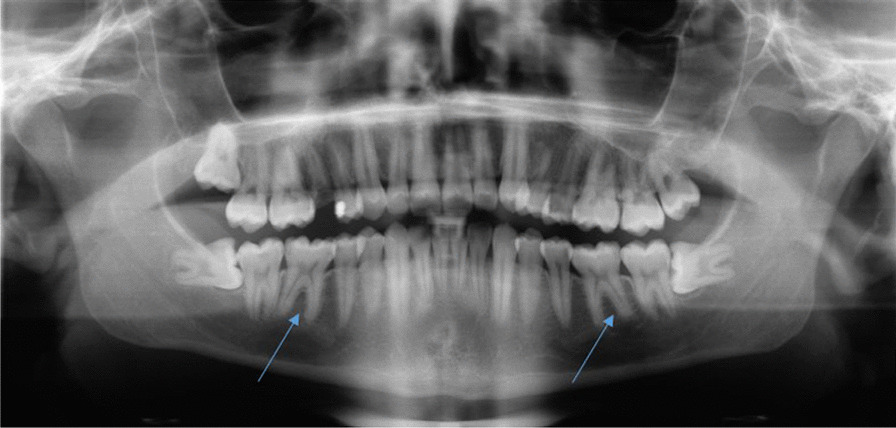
Routine X-Ray taken in January 2019 by the primary dentist. Tooth 36 and 46 without any apical osteolysis before the intensive of bruxism started. Both teeth have no signs of caries and no fillings are visible
